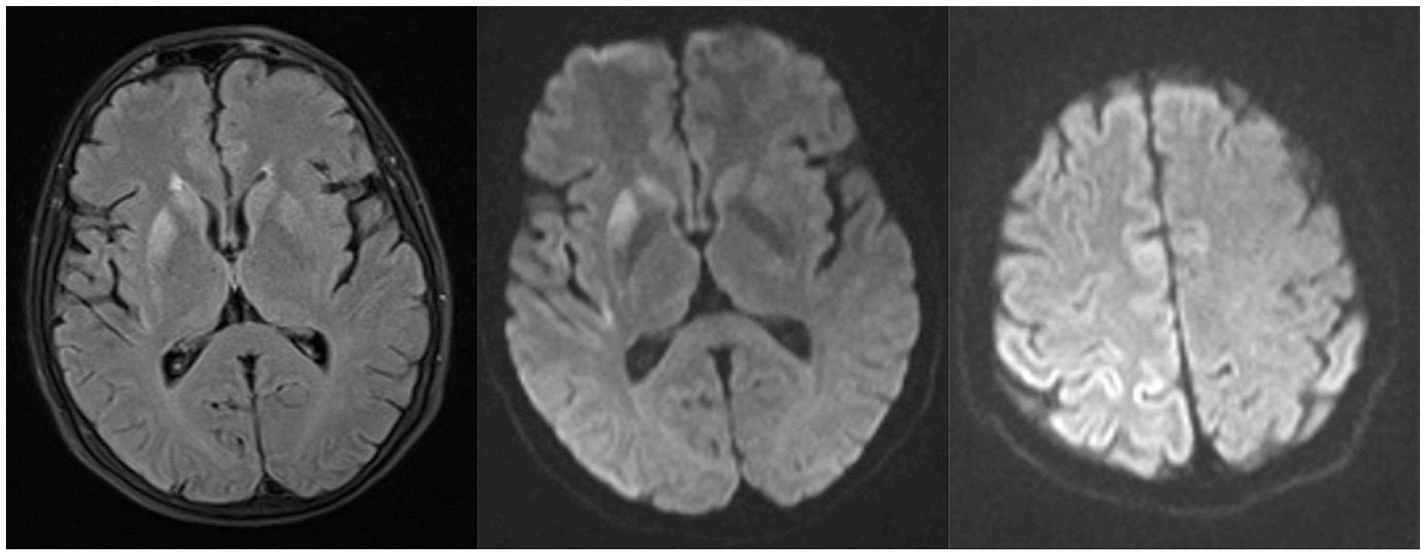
Brain MRI showing faint hyperintensity on Fluid Attenuated Inversion Recovery (FLAIR) images and positive diffusion-weighted (DWI) signal in the right lenticular and caudate nuclei, posterior insular and fronto-parietal cortex, without cortical atrophy or gadolinum enhancement.
